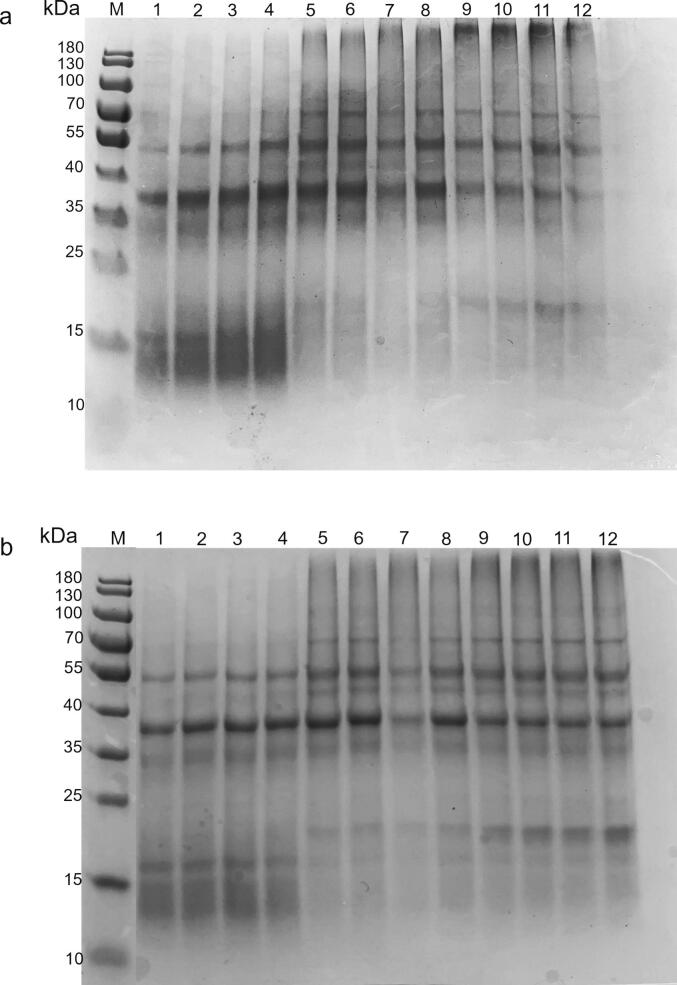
(a) Non-reducing and (b) reducing SDS-PAGE profiles of control and ultrasound-treated (20 kHz, 40 kHz, and 20 + 40 kHz) FPIs. Lane M: molecular weight standards, Lane 1–4: FPI-control, FPI-20, FPI-40, and FPI-20 + 40 at pH 3, Lane 5–8: FPI-control, FPI-20, FPI-40, and FPI-20 + 40 at pH 7, Lane 9–12: FPI-control, FPI-20, FPI-40, and FPI-20 + 40 at pH 9.
